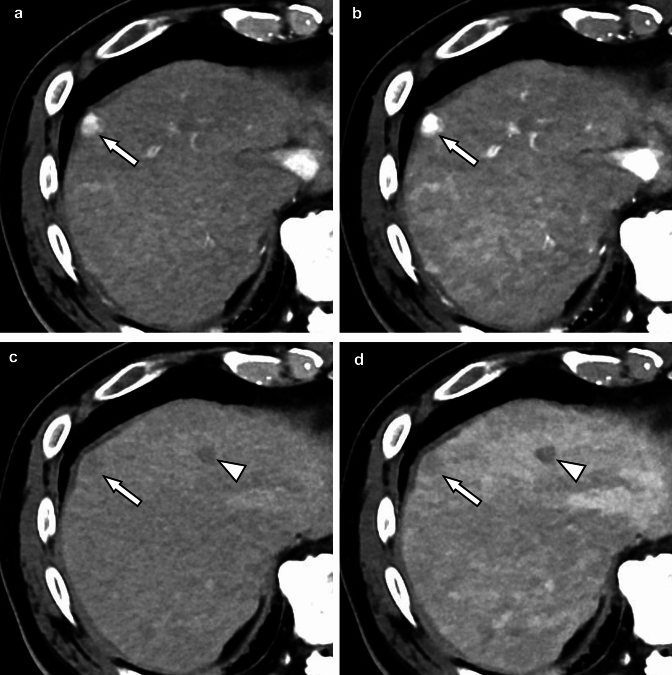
Contrast-enhanced photon-counting CT images during arterial dominant phase (A, B) and equilibrium phase (C, D) at 70 (A, C) and 50 keV (B, D). Arterial enhancement and washout pattern of HCC are better visualized on 50 keV images (B, D) than on 70 keV images (A, C) in the arterial and equilibrium phases, respectively (arrows). The hypovascular nodule (arrowheads: suspected dysplastic nodule) is also more clearly delineated on the 50 keV image (B) than on the 70 keV image (D)
